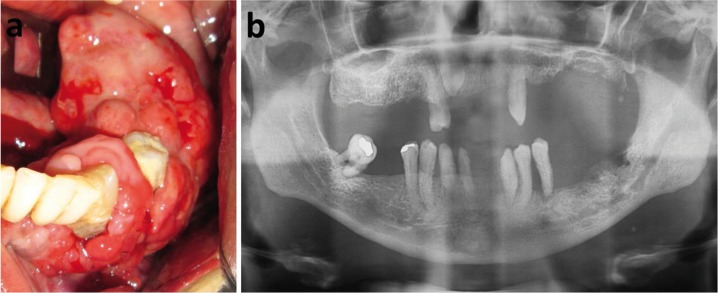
a) Clinical features of the desmoplastic fibroblastoma, extending from the retromolar region to the element 33. The lesion has lobulated surface and is covered by healthy mucosa with erythematous areas. Patient presented periodontal disease and poor oral hygiene. b) Panoramic radiograph shows irregular alveolar bone loss.
